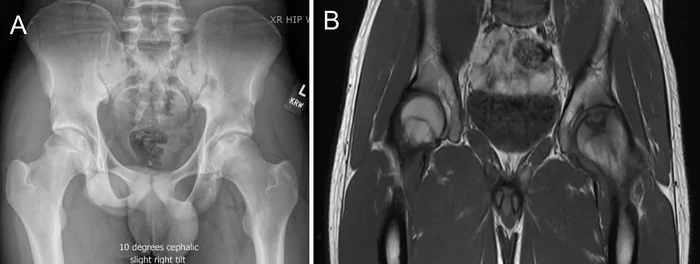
Preoperative imaging demonstrates hip pathology. (A) Pelvic 10° cephalic tilt bilateral hip radiograph shows a 1.5-cm left superior femoral head osteochondral defect and irregularity of the left humeral head. (B) Coronal T2-weighted, fat suppression bilateral hip magnetic resonance image shows left femoral head serpiginous double-line sign, indicative of avascular necrosis, cortical collapse, labral tear, subchondral edema, and hip effusion.
